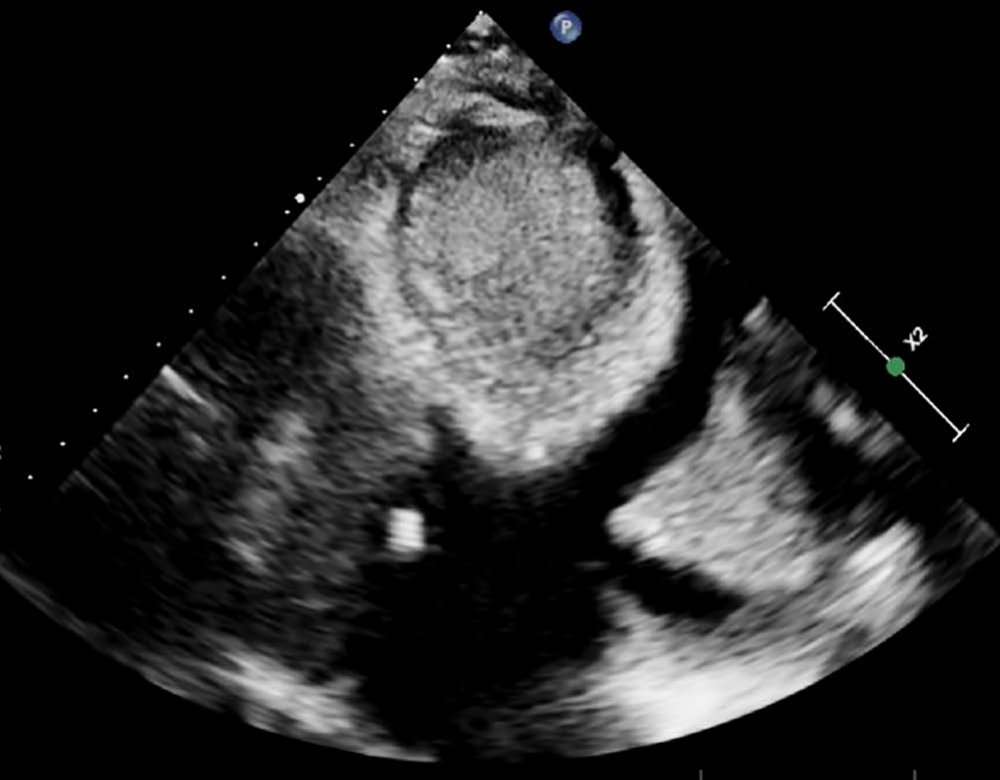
Transthoracic echocardiography demonstrating left ventricular apical obliteration.
